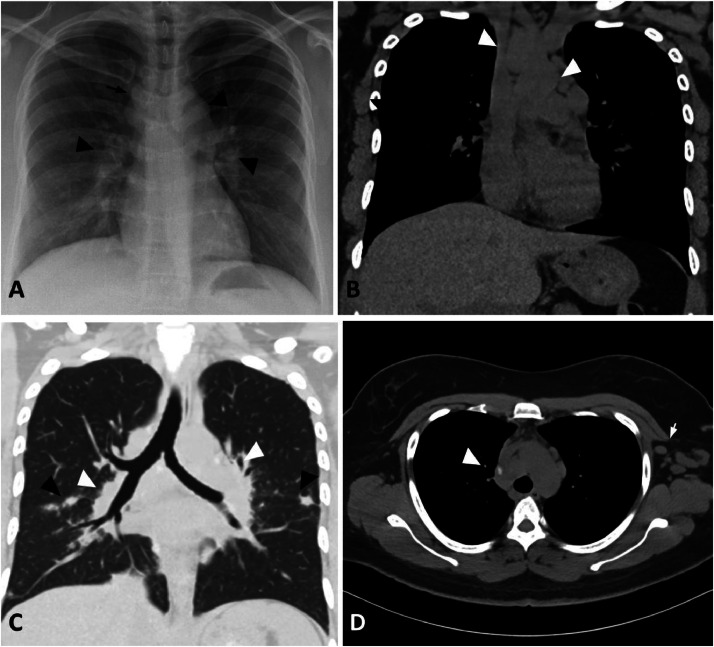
(A) Posteroanterior Radiograph of the chest shows thickening of the right paratracheal stripe (black arrow) with lobular contour of the aortic knob and lung hila (black arrow heads) representing mediastinal and hilar adenopathy. (B) Coronal CT image of the chest without contrast in soft tissue window at the level of the mid-axilla demonstrates prominent mediastinal lymph nodes (white arrowheads). (C) Coronal CT image of the chest without contrast in lung window at the level of the carina demonstrates multiple peri-fissural and subpleural nodules (black arrowheads) and hilar lymph nodes with adjacent central nodules (white arrowheads). (D) Axial CT image of the chest without contrast in soft tissue window at the level of the mid trachea shows calcified mediastinal lymph nodes (white arrowhead) and prominent axillary lymph nodes (white arrow).
